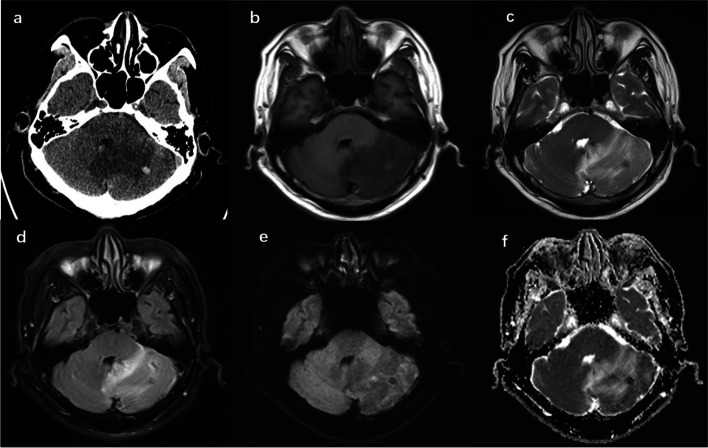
a CT imaging showing a lesion in the Left cerebellar hemisphere, with a large low-density area in the left cerebellar hemisphere and vermian and a small, round-like, and high-density area in the left cerebellar hemisphere; b MRI imaging showing hypointense on T1WI; c MRI imaging showing hyperintense on T2WI; d MRI imaging showing FLAIR; e MRI imaging showing slightly hypointense signal on DWI; and f MRI imaging showing hyperintense on ADC
